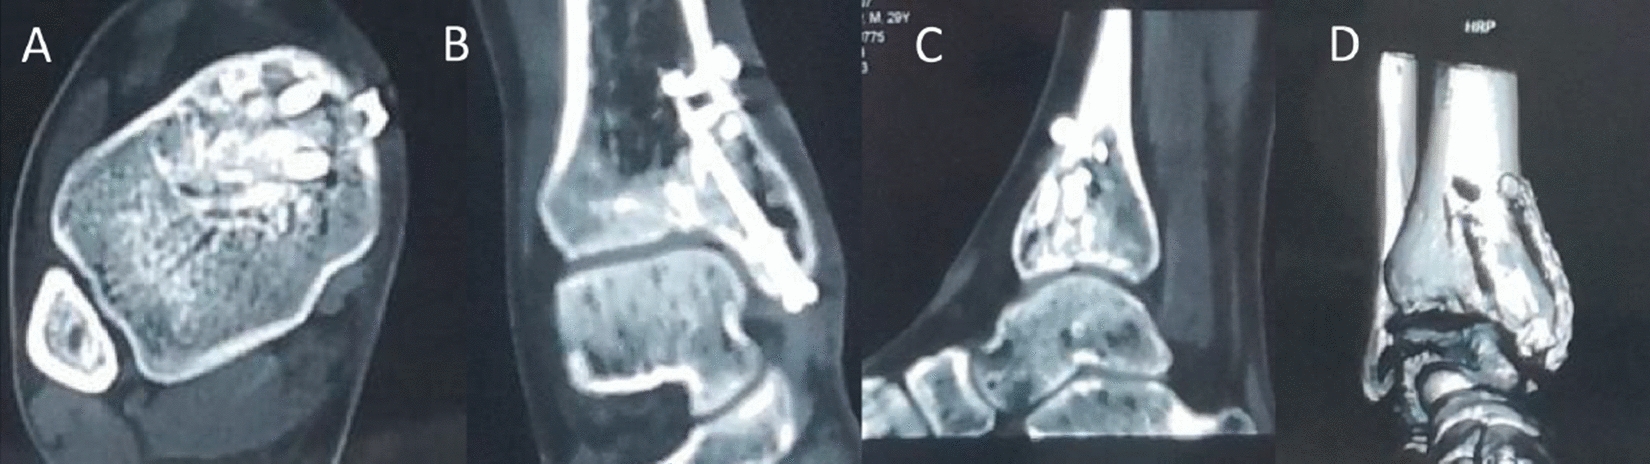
CT 1 year review of the ankle joint after surgery (a–d): resection of the distal right tibia lesion after bone graft fusion with plate and screw internal fixation. The bone graft was filled and fused well. Steel plate and screw fixed firmly, no fracture, bone nonunion, etc
